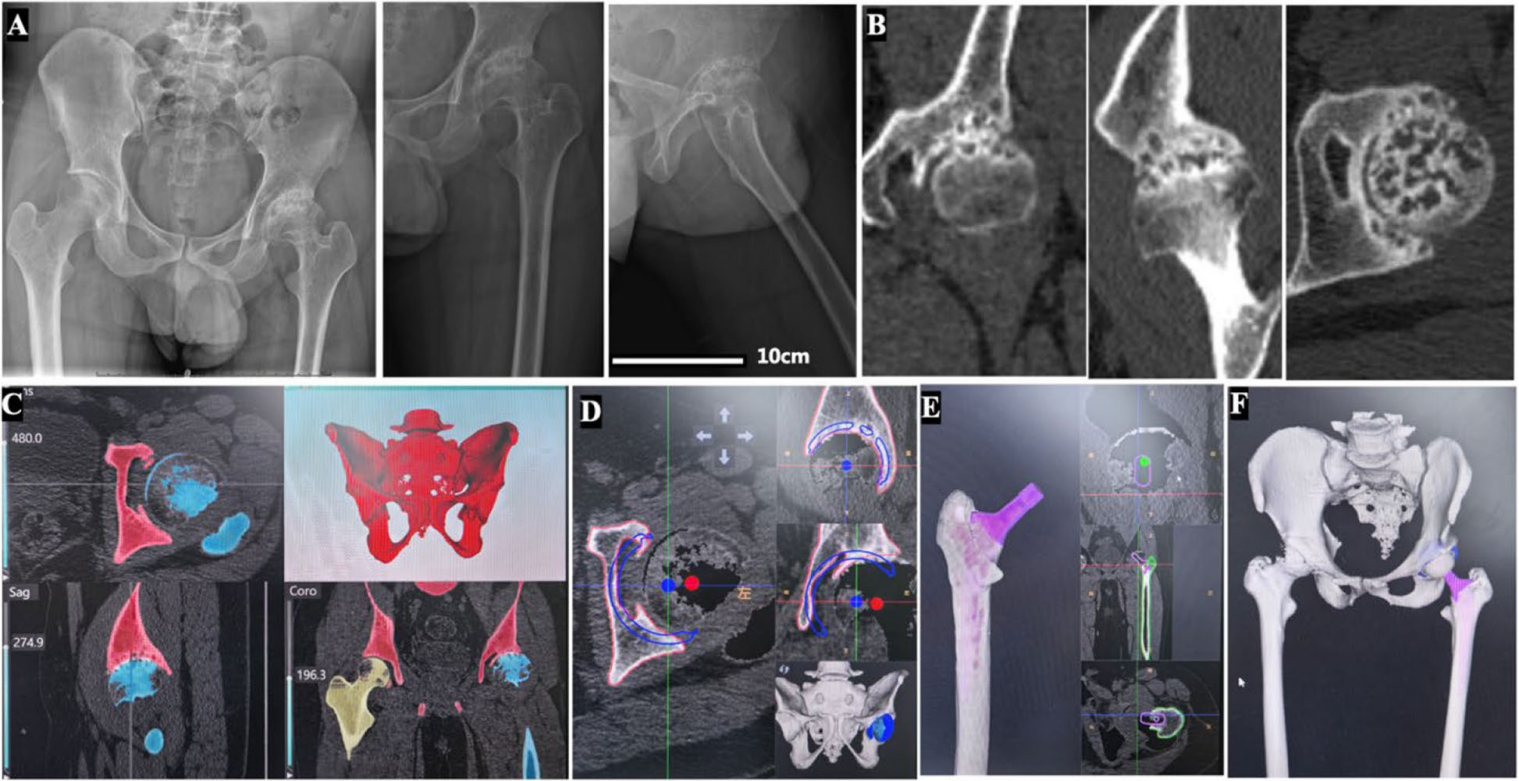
Preoperative imaging and robotic planning. A. Preoperative X-ray radiographs: anteroposterior pelvic view (left), anteroposterior left hip view (middle), and lateral left hip view (right) X-rays. The left hip joint shows collapse and deformation of the femoral head with a narrowed joint space and acetabular wear. B. Preoperative CT scans: Coronal (left), sagittal (middle), and axial (right) planes. Reveals left femoral head and acetabular deformity with cystic changes. C. Preoperative robotic system-assisted (RA) CT segmentation. D. Preoperative RA prosthesis planning of the acetabular component based on CT scans. E. Preoperative RA prosthesis planning of the femoral component based on CT scans. F. Preoperative RA prosthesis planning via 3D imaging
